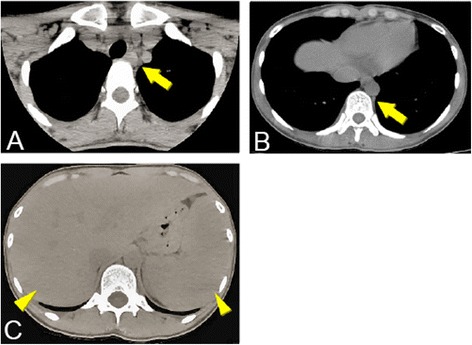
Computed tomography (CT) from cervical to abdomen: A)
